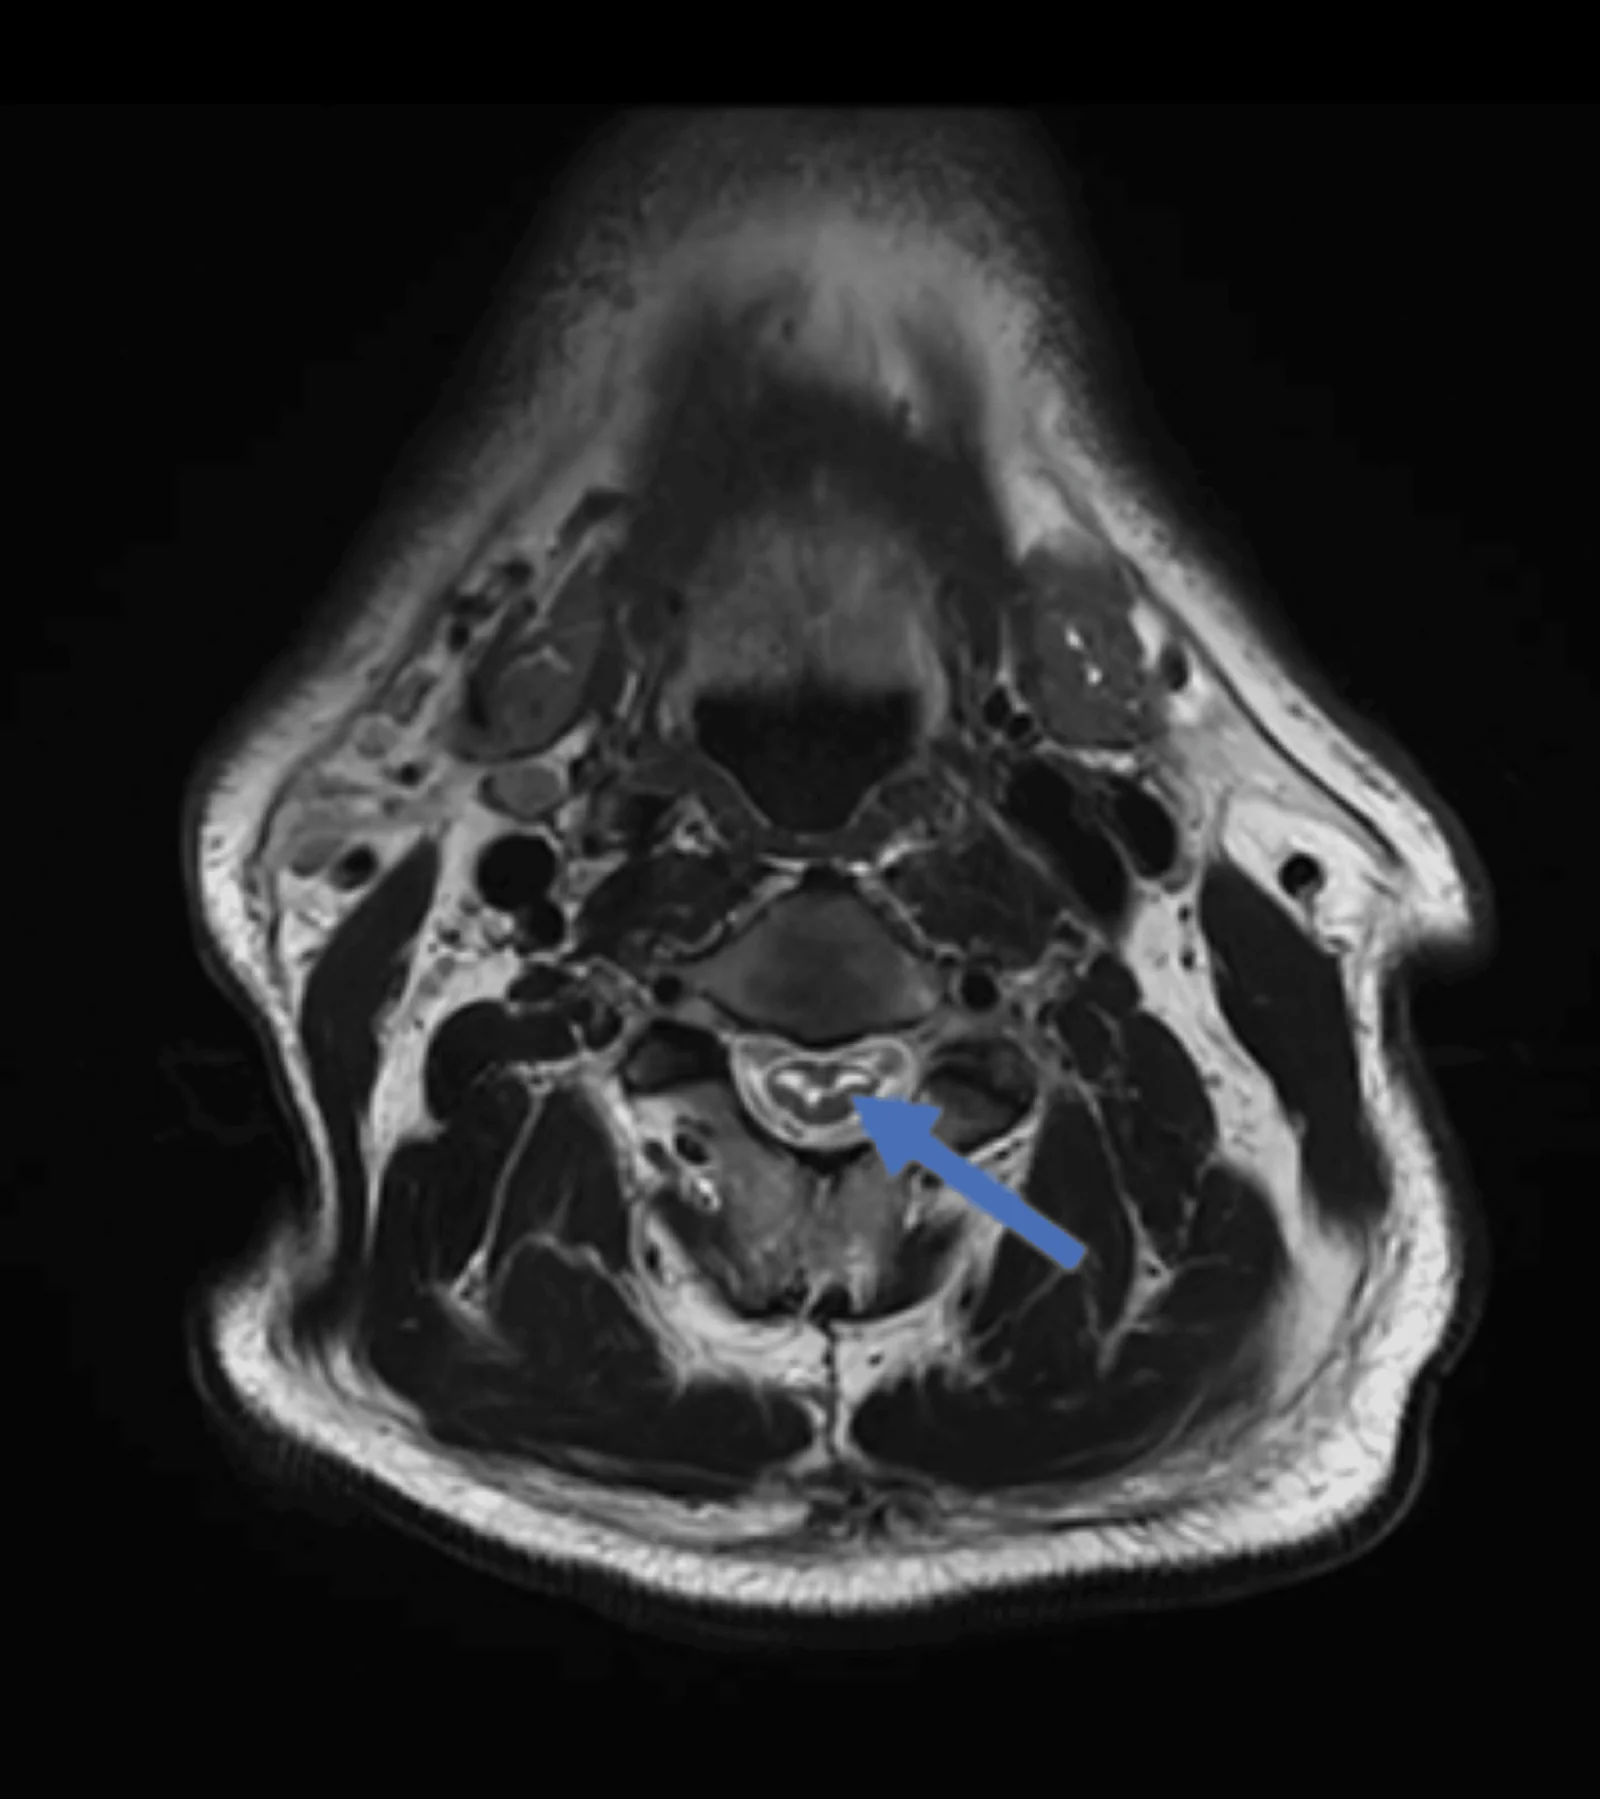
Axial T2-weighted MRI at the C2-C3 level Axial T2-weighted MRI at the C2-C3 level showing central intramedullary T2 hyperintensity (arrow), consistent with spinal cord signal changes. MRI: magnetic resonance imaging
